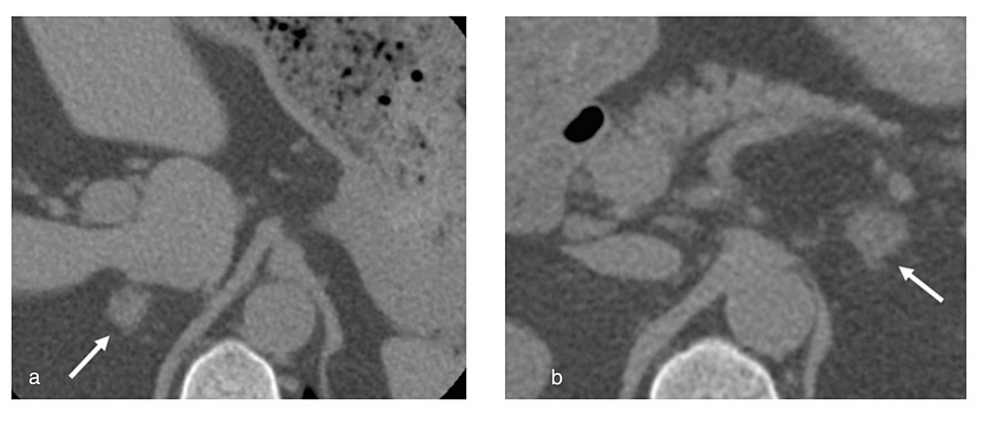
Adrenal CT showing interval decrease in the size of adrenal hemorrhage bilaterally compared to the initial presentation
